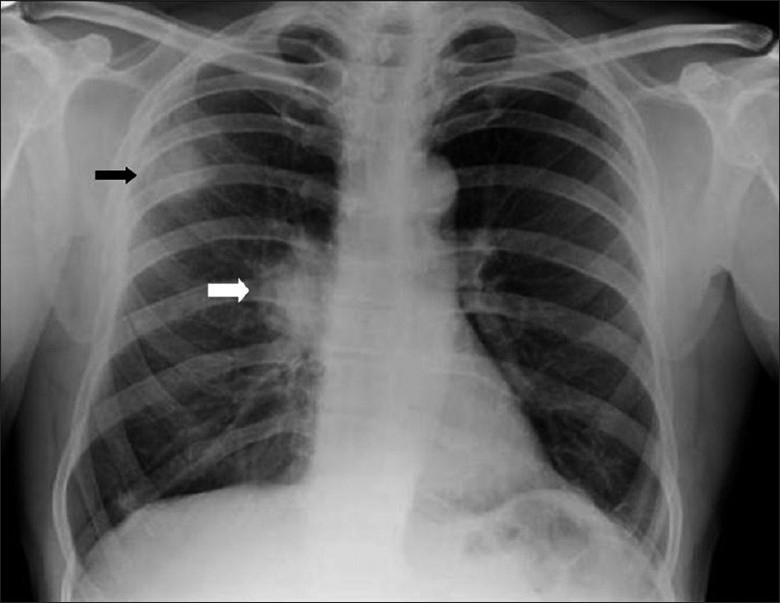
Bronchogenic carcinoma. Radiographic appearances are similar to ordinary lung cancer, except that lesions tend to be more peripheral and in the upper lobes as in this case (black arrow). Note the right hilar lymphadenopathy (white arrow)
